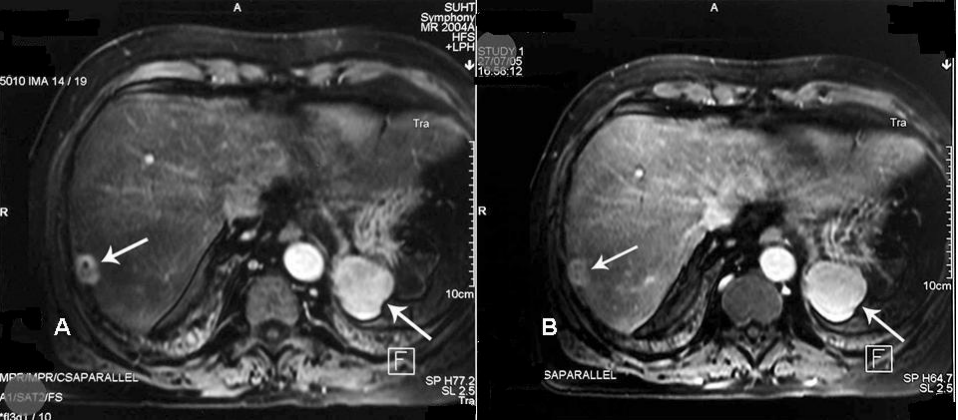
Arterial (A) and portal venous phase (B) of IV Gadlinium enhanced axial MRI images demonstrating a solitary 2 cm hypervascular nodule in segment VII (Arrow). A 4.5 cm nodule with similar enhancement characteristics is also noted in the left upper quadrant (Arrow).
